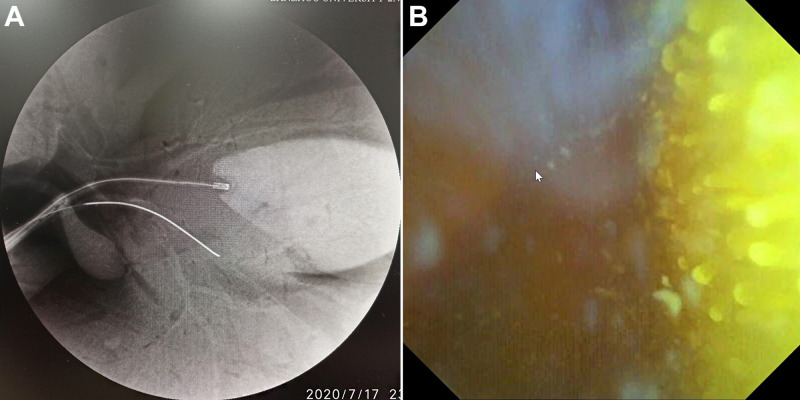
Catheter Entering Gallbladder Under X-ray (A) and Villi inside Gallbladder (B).
